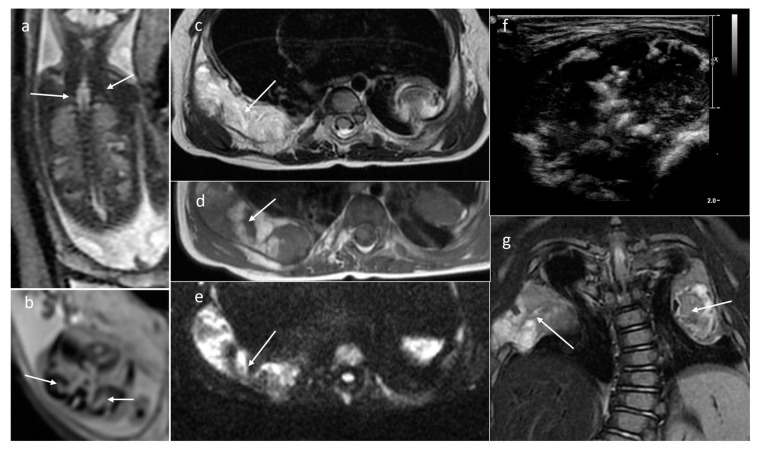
Mesenchymal hamartomas of the chest wall. Bilateral lesions of the ribs in a fetus. (a) Coronal SSFSE/T2WI; (b) axial GRE EPI. Follow-up MRI after birth. (c) Axial T2WI; (d) axial T1WI; (e) DWI; (f) US transverse section; (g) coronal T2WI.
